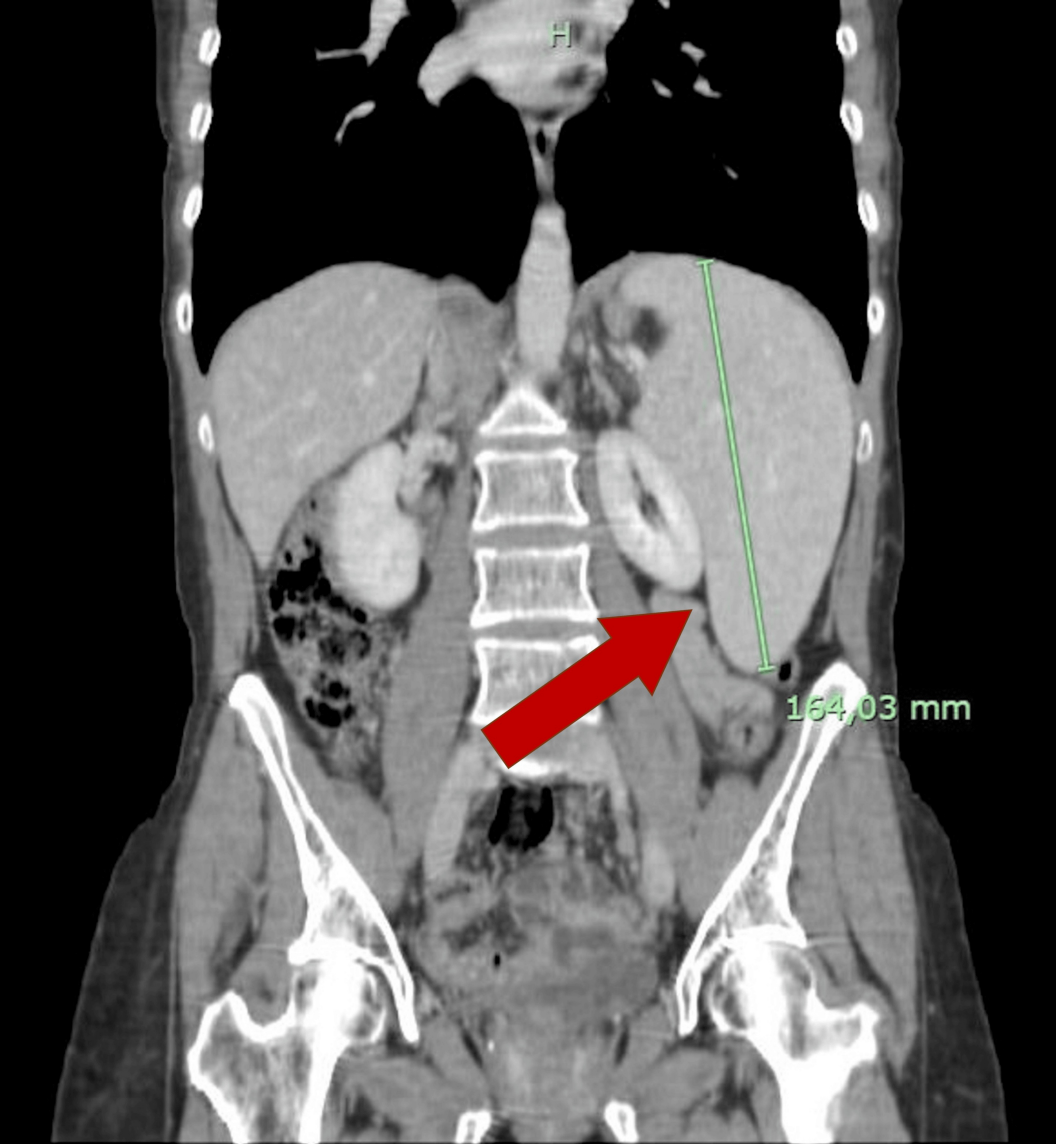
CT scan 19 months after T-DM1 treatment was started, showing de novo splenomegaly (red arrow).
